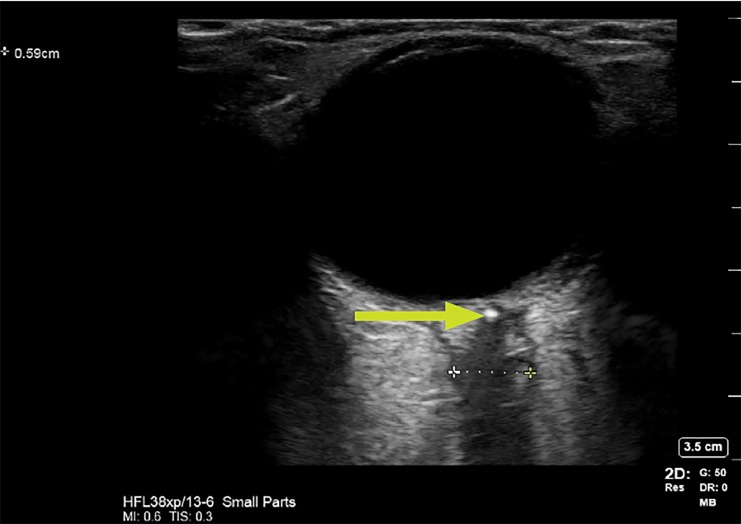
Point-of-care ultrasound of left eye with linear probe small-parts setting, demonstrating likely clot (yellow arrow) and edematous optic nerve (measured at 3.5cm).
